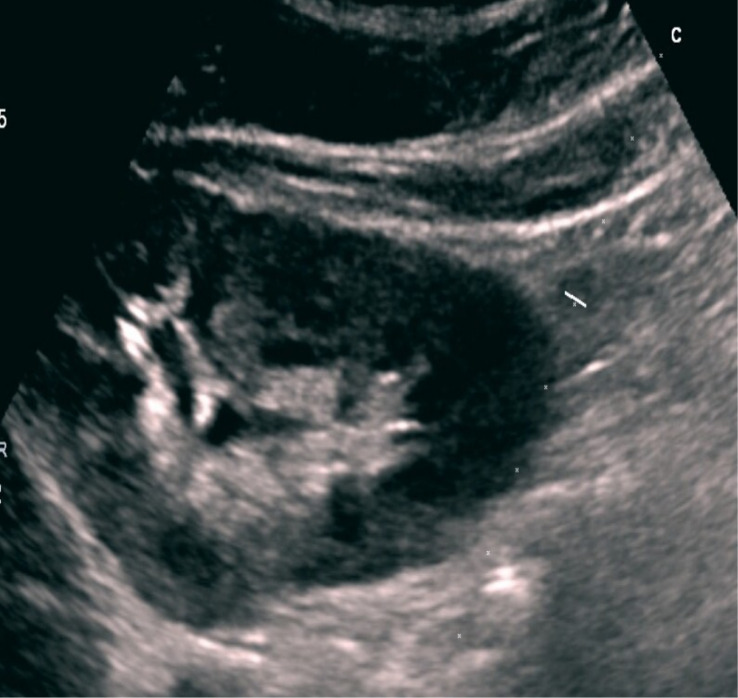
Ultrasound-guided needle placement (right kidney lower pole).
